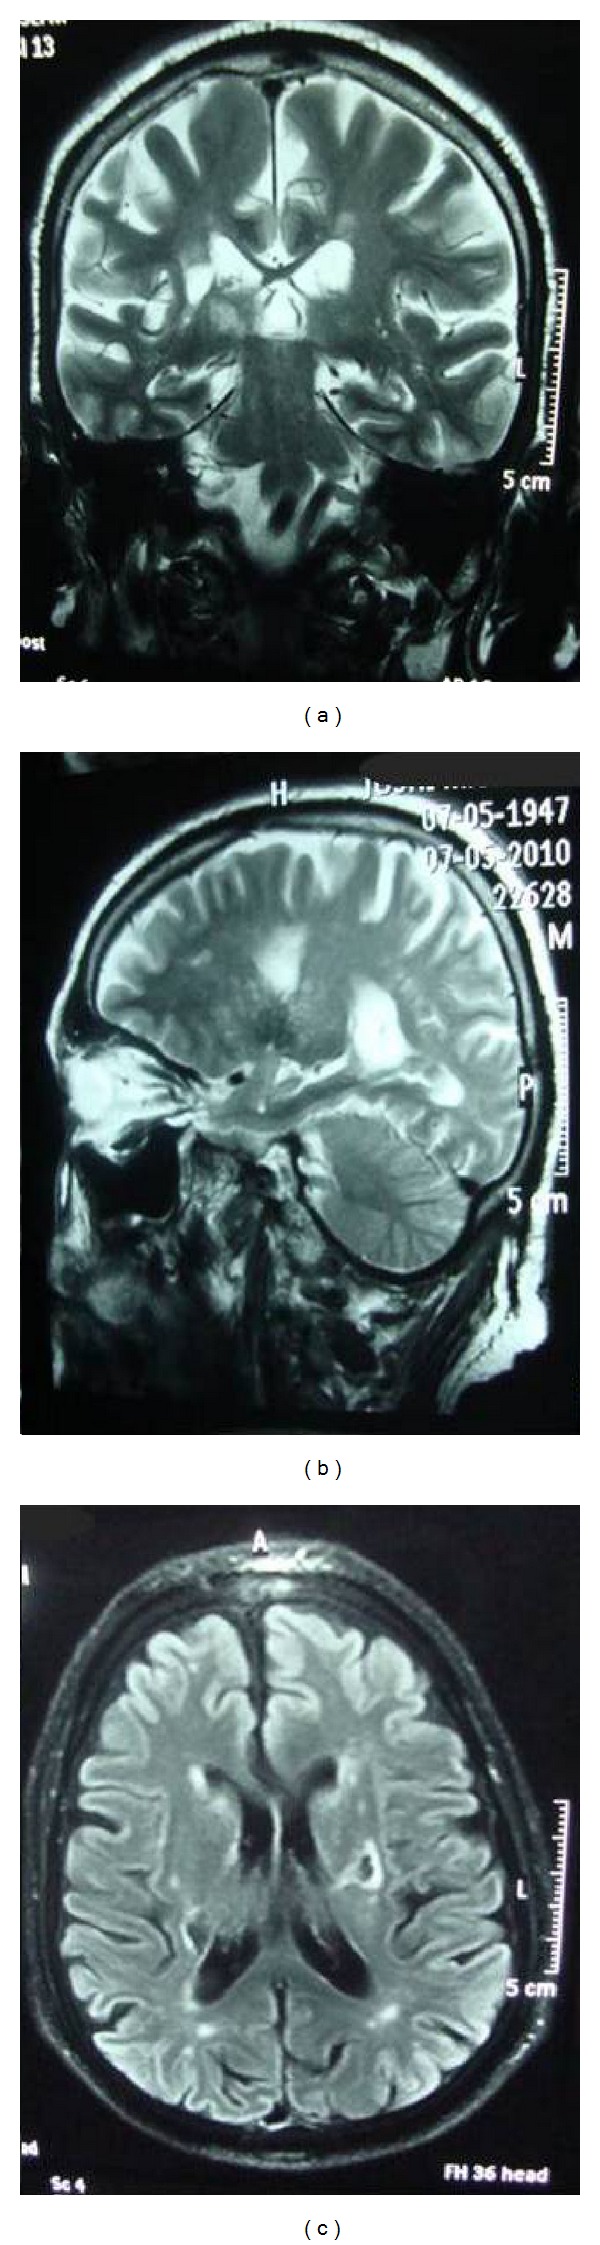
Magnetic resonance imaging of the brain; frontal, sagittal, and transverse sections showing an acute nonhemorrhagic right middle cerebral artery territory infarct with the putamen extending to the corona radiate, 12 hours after-stroke.
